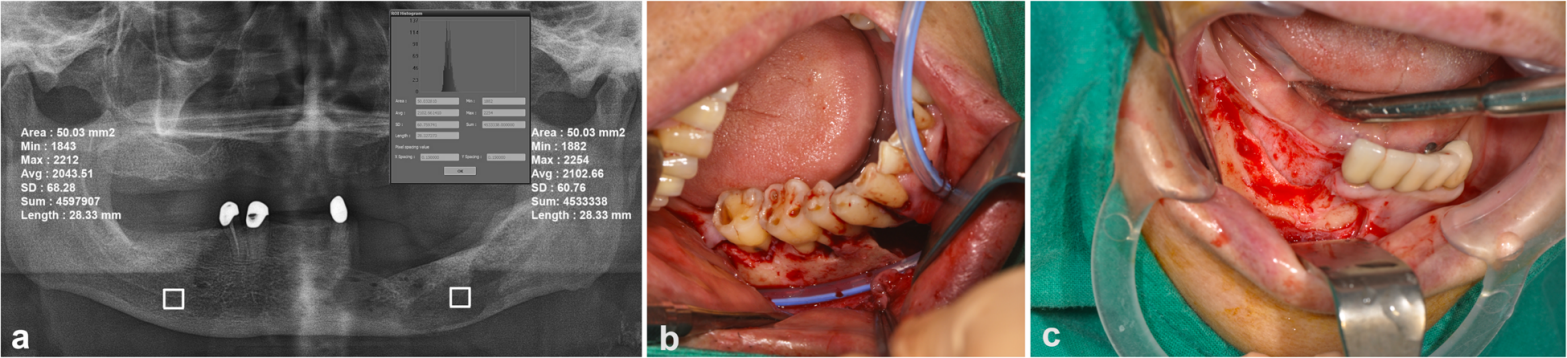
The radiographic bone density expressed as grayscale values (GSV) were measured by using the easy digitalized panoramic analysis method. Rectangle annotation analysis of INFINITT PACS® (INFINITT Healthcare, Seoul, Korea) was used for measuring the radiographic bone density GSV in suppurative OM as shown in patient No.7. A standard rectangular lesion area of approximately 50 mm^2^ was defined as the region of interest, designated at the center of the OM lesion. The bone density of the contralateral bone in the healthy region was also measured as a reference value to determine the absolute value (a). Intraoperative view of decompressive drain insertion intraorally following surgical management in suppurative type OM with drain (b). Intraoperative view of surgical debridement in sclerosing OM without drain (c)
